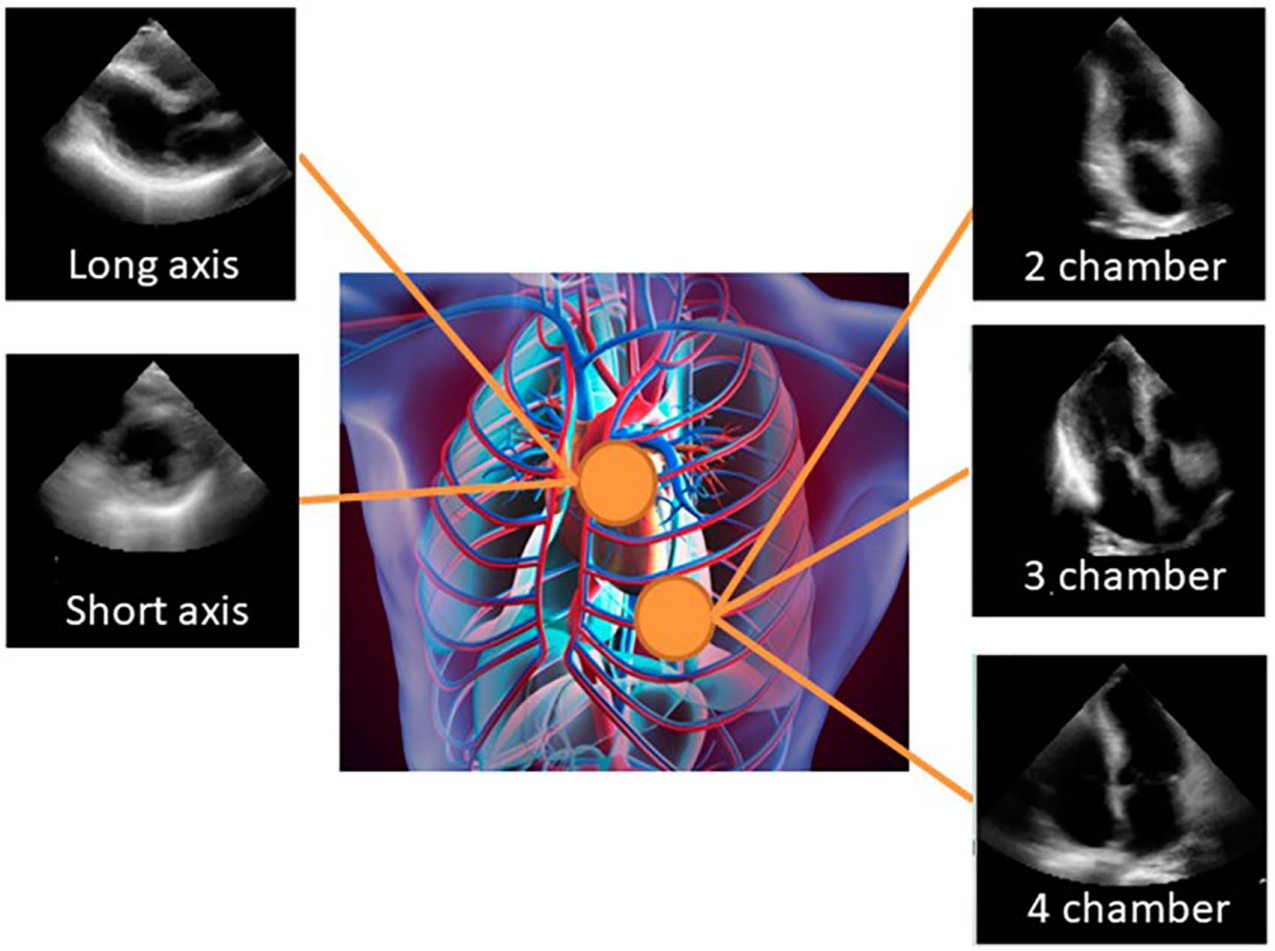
Sample of typical cardiac views: apical 2-chamber, apical 4-chamber, apical 3-chamber, parasternal long axis (PLAX), and parasternal short axis [PSAX; image from (28)].
